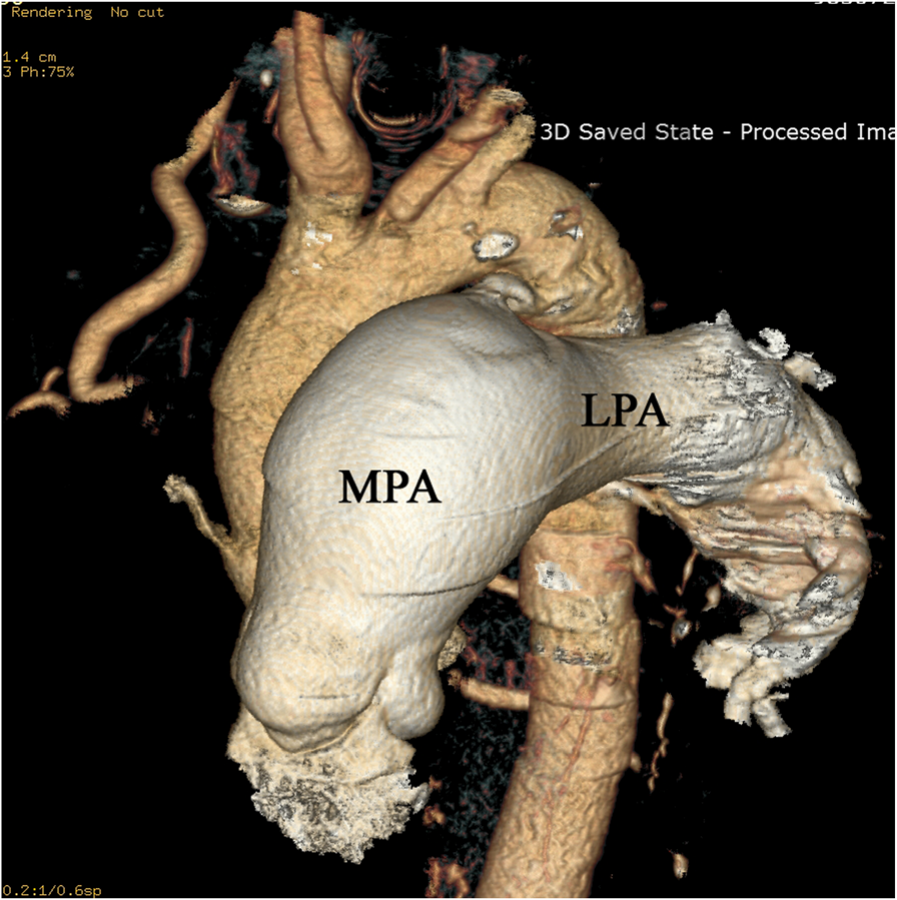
CT angiography showing agenesis of right pulmonary artery. Aneurysmal dilatation of main pulmonary artery (MPA) and left pulmonary artery (LPA) and evidence of PDA
